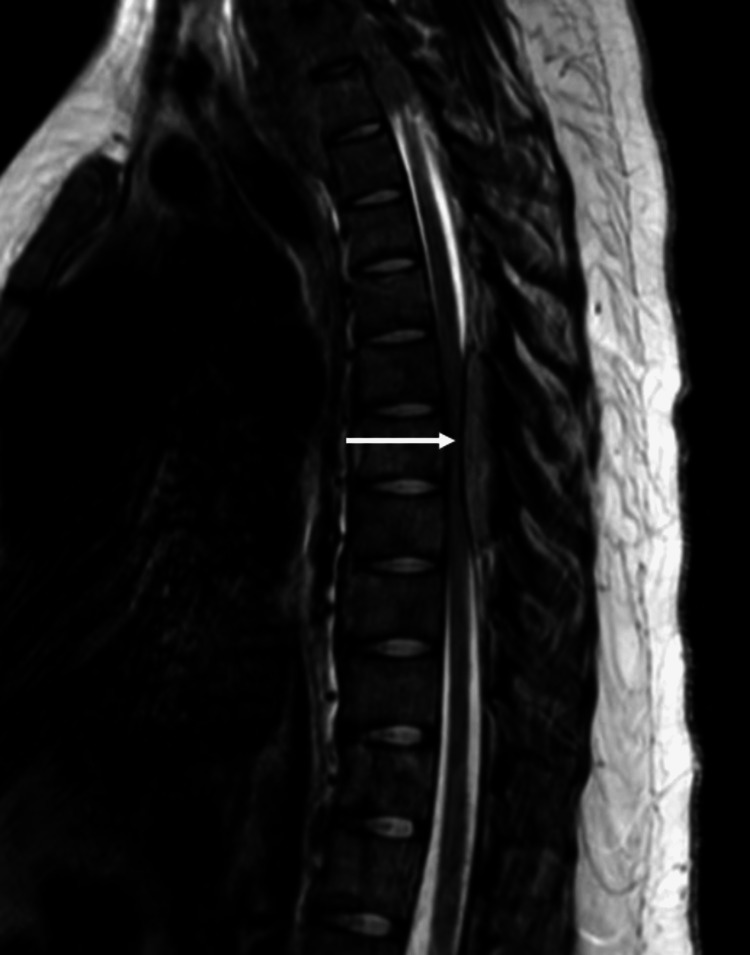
Sagittal T2 STIR MRI of the thoracic spine. MRI of the thoracic spine revealed a soft tissue tumor (white arrow) in the spinal canal at the T6-T9 level compressing the mid-thoracic spinal cord. STIR: short inversion time inversion recovery
